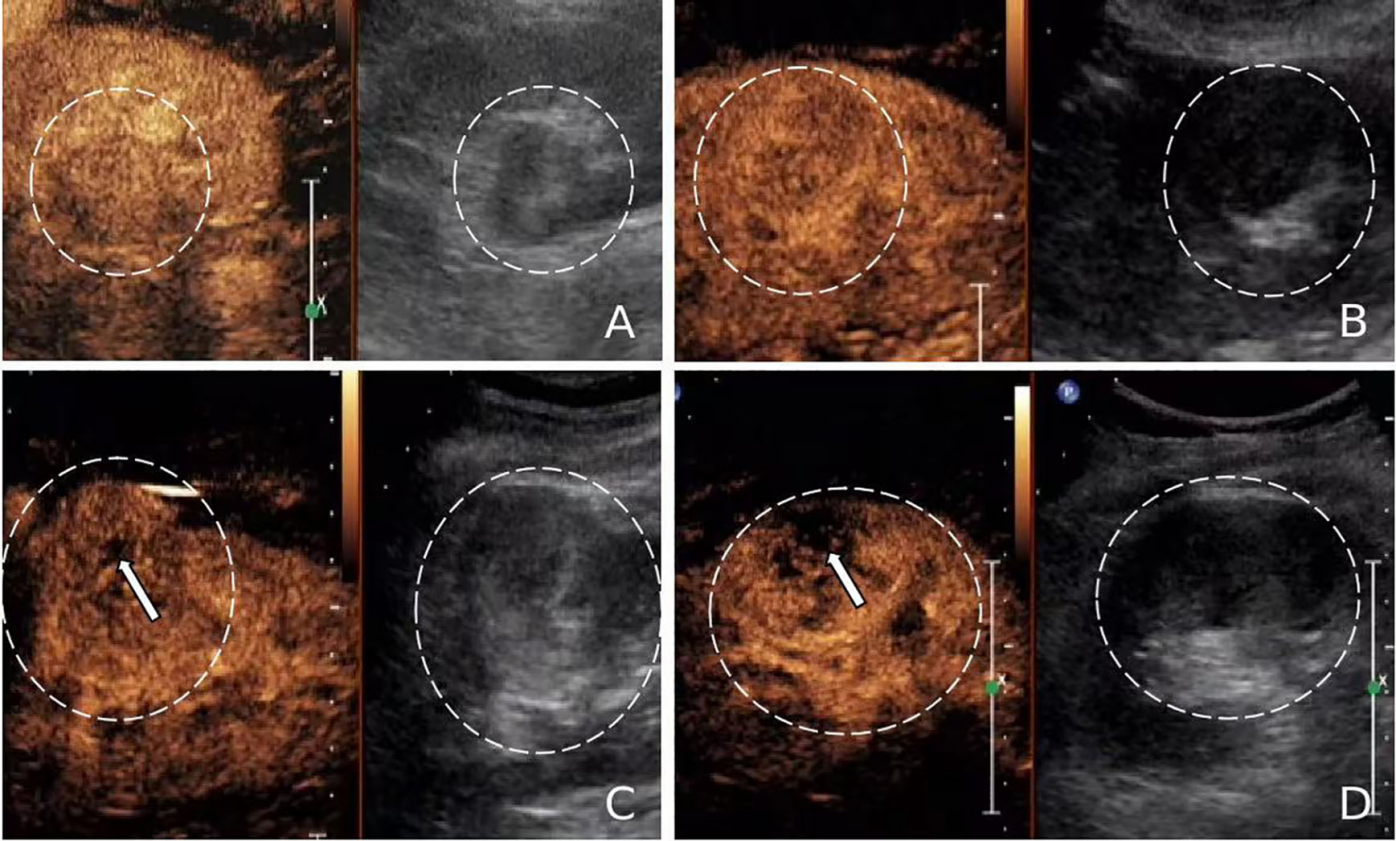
(A, B) Contrast-enhanced ultrasound (CEUS) images of fat-poor angiomyolipoma(AML) (circled) showing uniform enhancement without unenhanced areas. (C, D) CEUS images of renal cell carcinoma (RCC) (circled) exhibiting heterogeneous enhancement with unenhanced areas (arrows).
